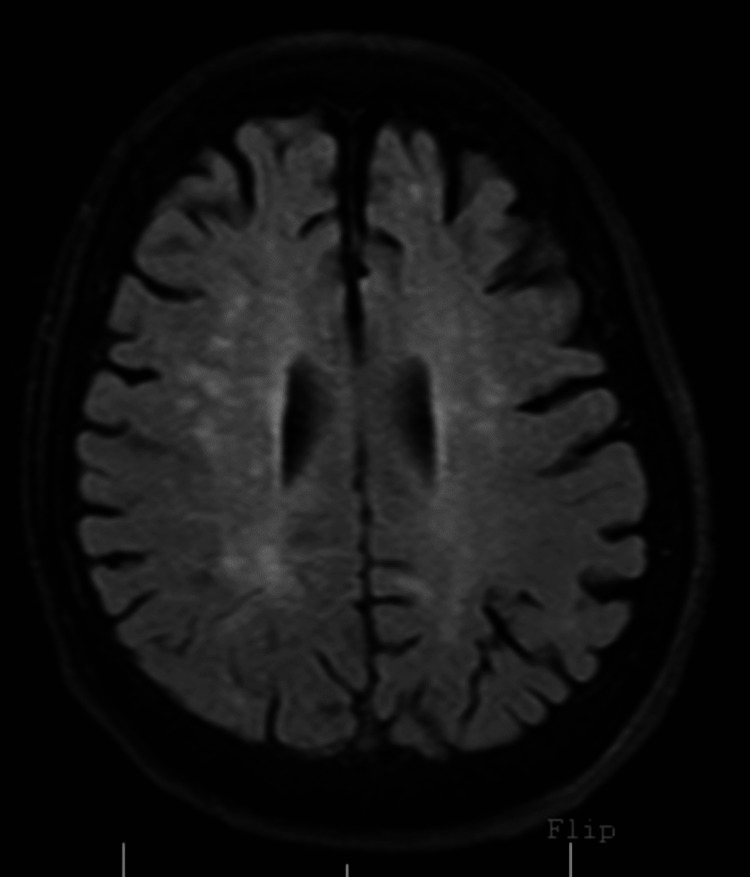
MRI brain showed moderate chronic microvascular ischemic changes. MRI: magnetic resonance imaging
